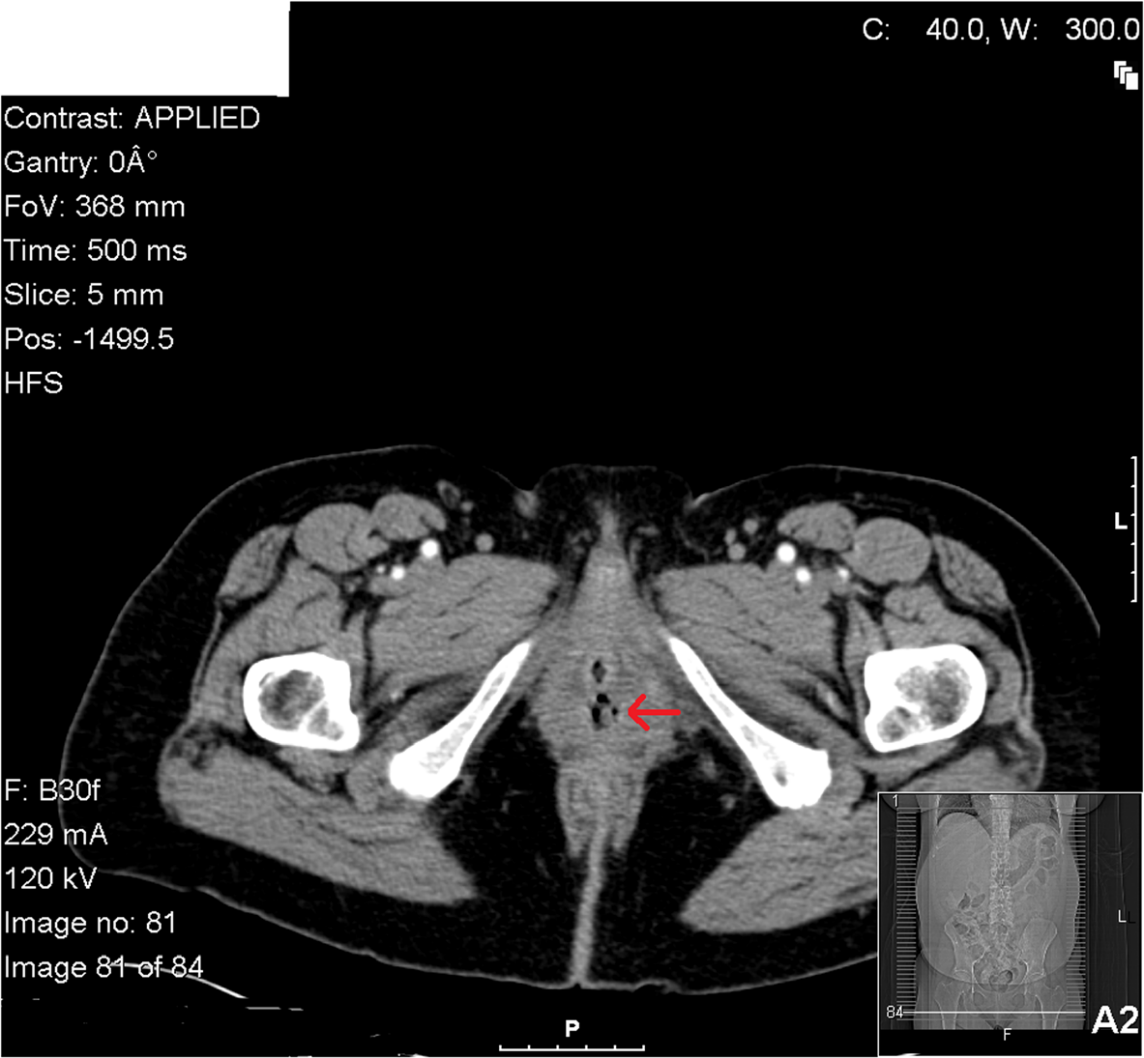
Computed tomography scan of urethral diverticulum. Red arrow indicates urethral diverticulum.
